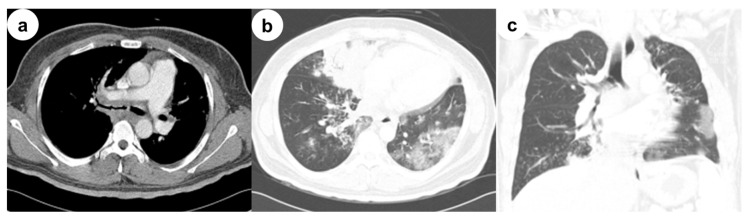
Initial contrast chest computed tomography (CT). (a) Selected axial image of the soft-tissue window on chest CT shows the thickening of the right main bronchus, a peribronchial soft-tissue lesion and lymphadenopathy; (b) Selected axial and (c) coronal images of the lung window on chest CT shows consolidation in the right middle lobe, diffuse and patchy densities with focal consolidations involving both lower lobes, and thickening of the right main and intermedius bronchi.
